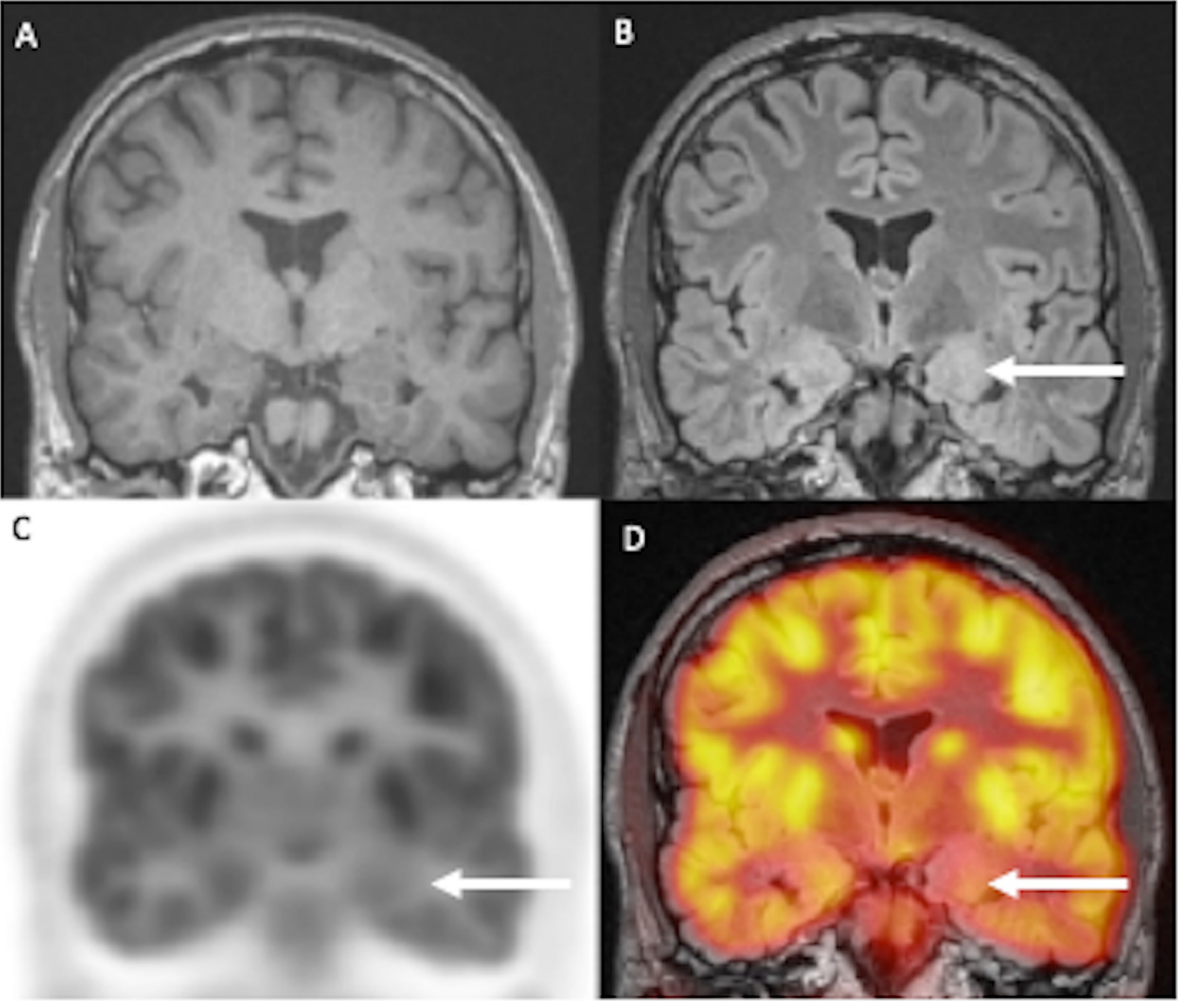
21-year old female patient with swelling and FLAIR hyperintensity of the left amygdala (A, B). PET shows corresponding glucose hypometabolism (C, D).
